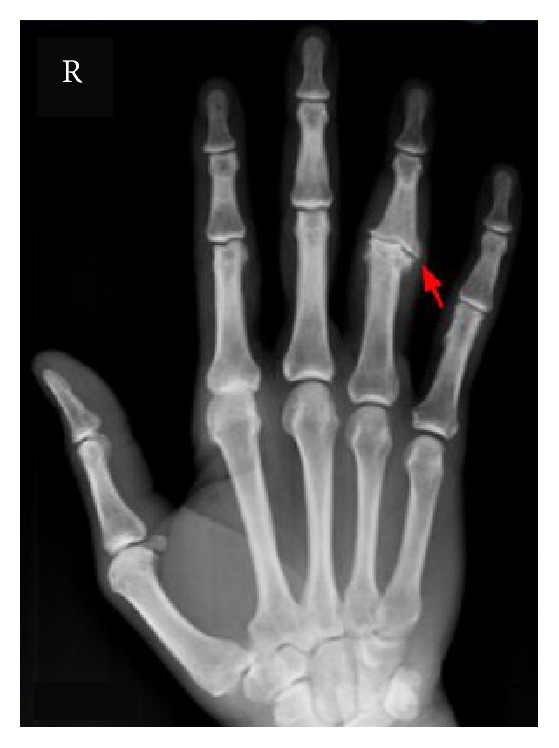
X-ray of right hand, severe degenerative changes at the fourth PIP joint with medial subluxation of the middle phalanx, and periosteal reaction.
